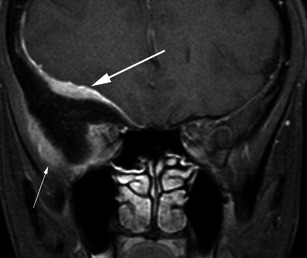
Post-contrast coronal T1-weighted sequence in a 33-year-old woman who presents with right proptosis demonstrates extensive dural thickening and enhancement along the greater wing of sphenoid consistent with an en plaque meningioma (*large arrow*). The lesion results in marked hyperostosis of the greater wing of sphenoid with resultant indentation of the right temporal lobe. Further enhancing tumour is identified external to the calvarium between the greater wing of sphenoid and the temporalis muscle (*small arrow*)
